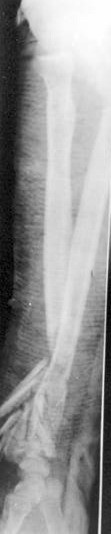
Lateral radiographs of his left forearm and wrist revealed the describing injury.
